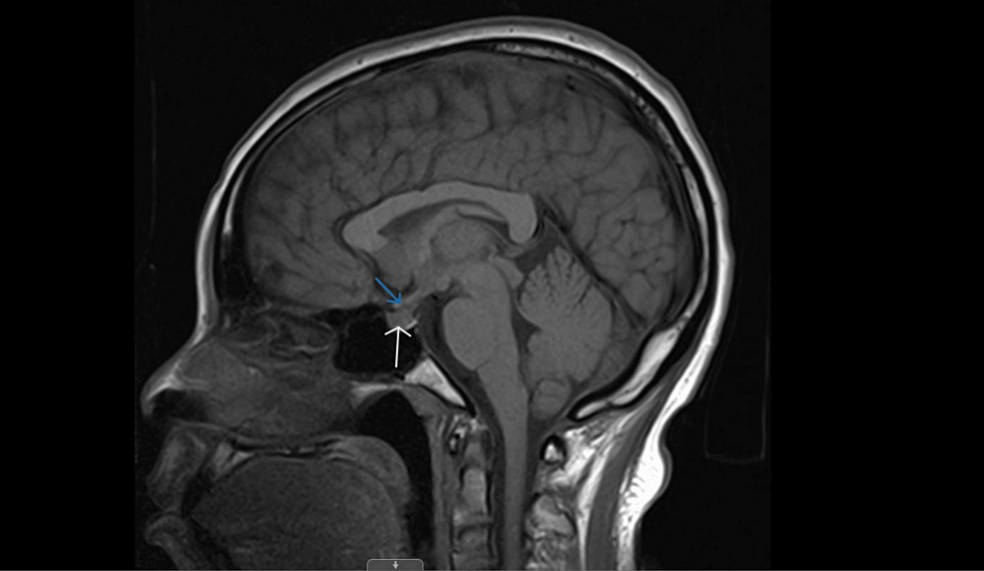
Brain MRI, sagittal section. White arrows: pituitary gland; blue arrows: pituitary stalk. MRI shows a slightly heterogeneous enhancement of the pituitary gland that measured 8 mm and slight thickening of the pituitary stalk inferiorly, features suggestive of hypophysitis. MRI, magnetic resonance imaging
